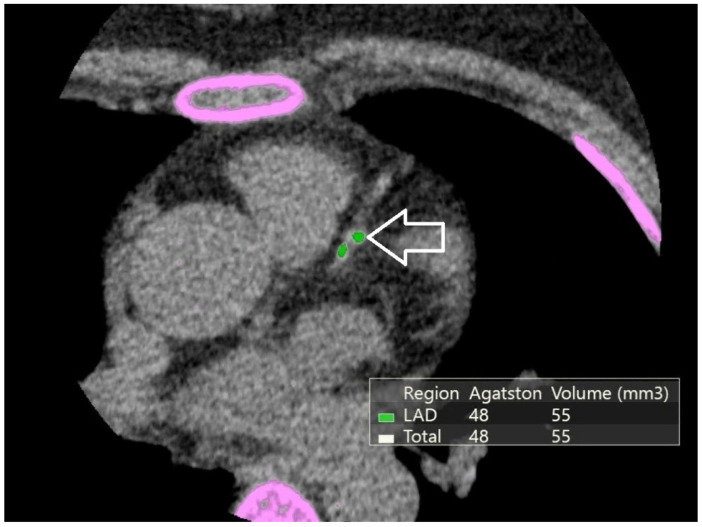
Non-contrast cardiac CT demonstrating coronary artery calcification. Pink overlays indicate software-based segmentation of calcified plaques contributing to Agatston score calculation. The white arrow highlights a representative calcified plaque in the left anterior descending (LAD) artery. The CAC score (Agatston method) serves as a quantitative marker of cumulative atherosclerotic burden.
